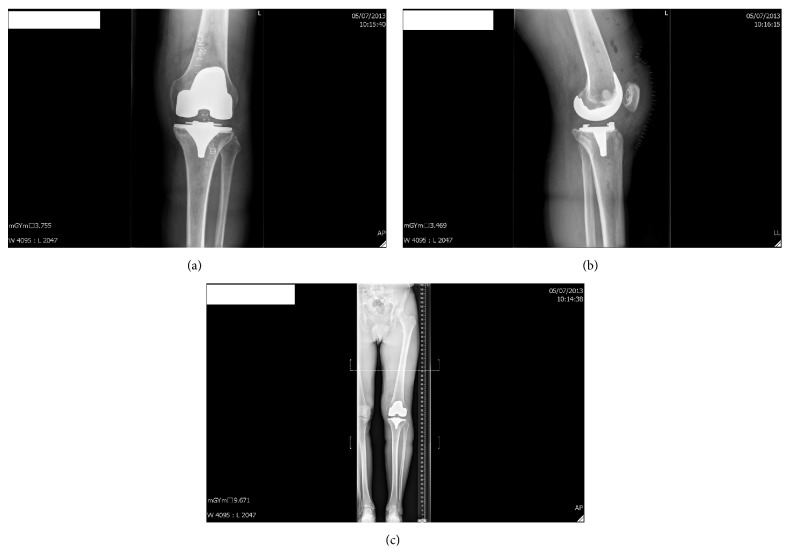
Anteroposterior (a), profile (b), and full leg view (c) X-rays of the knee after revision surgery.
